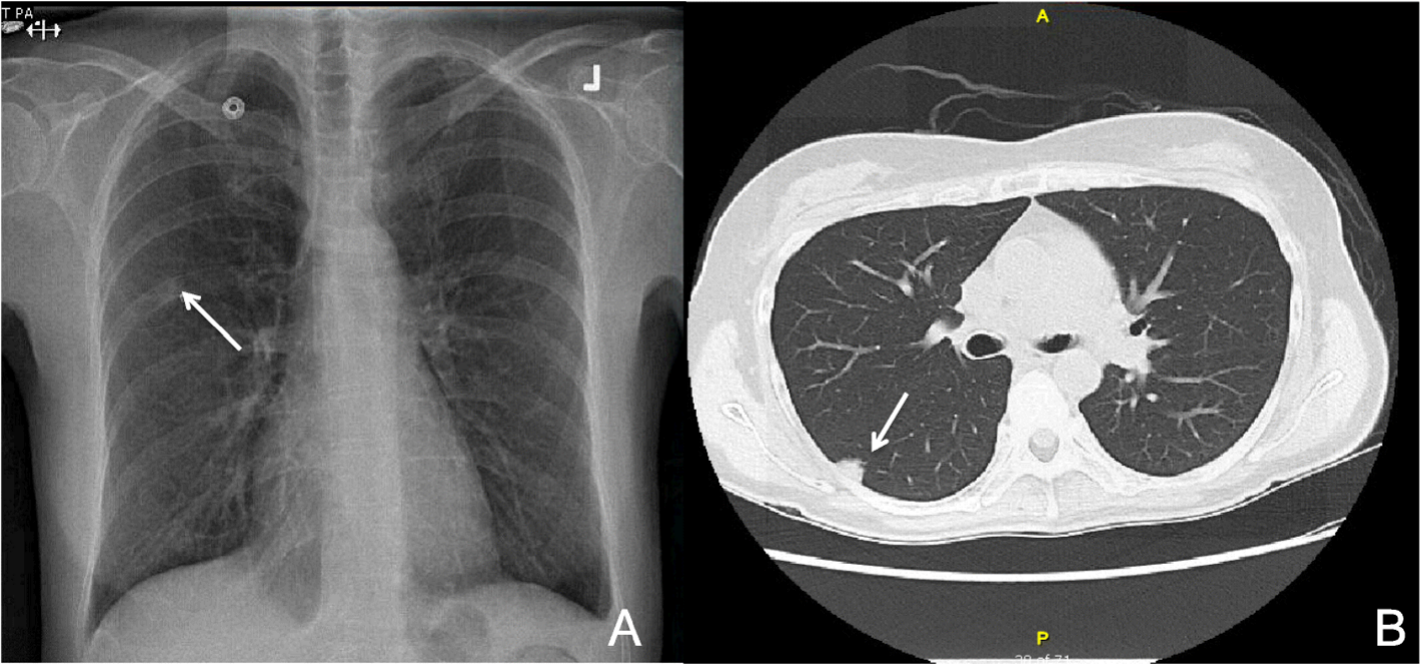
Chest radiograph demonstrating nodular opacification in the right upper lung (a). Chest computed tomography [3] scan demonstrating non-calcified and non-cavitating pleural-based lesion laterally in the superior segment of the right lower lobe measuring 14 × 11 mm (b)
